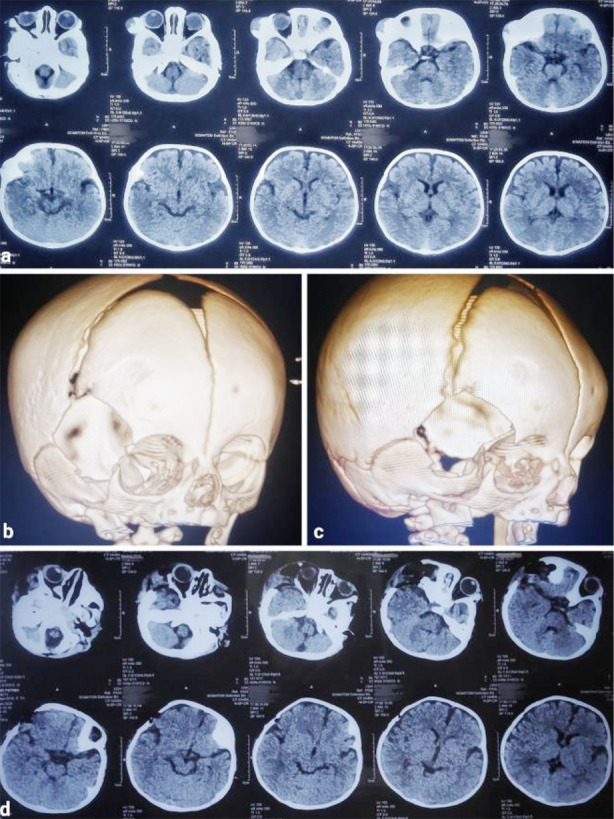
(a) Preoperative CT brain without contrast showing iso- to hypodense soft tissue swelling with surrounding hyperdense bone overgrowth of the lateral orbital wall and right temporal bone. (b & c) Postoperative CT with 3D skull reconstructions showing resection of the right frontotemporal bone and lateral orbital wall. (d) Postoperative CT brain without contrast showing complete resection.
